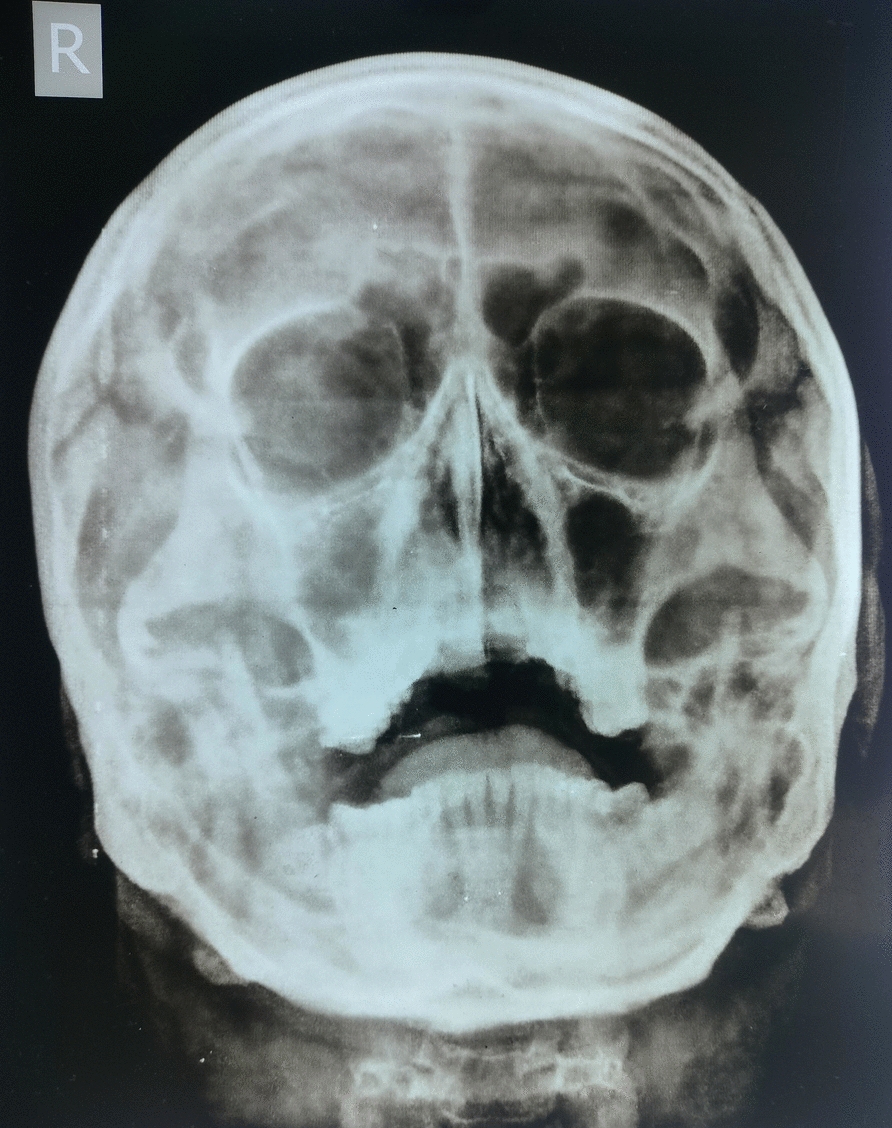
Initial paranasal sinus radiograph showing features suggestive of sinusitis. Water’s view paranasal sinus radiograph shows a midline nasal septum with mild diffuse haziness of the right maxillary sinus, consistent with mucosal thickening. The left maxillary sinus appears relatively clear. No bony erosion, destructive changes, or septal deviation are identified
